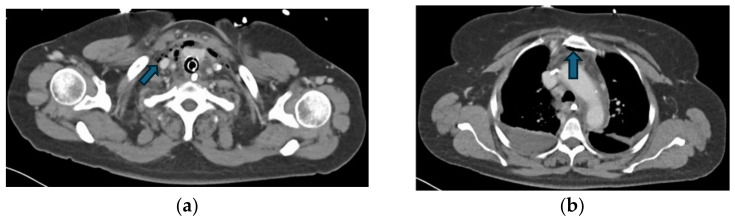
CT findings of the same patient presented in Figure 2, demonstrating the progression of DNM. (a) Gas inclusions (blue arrow) extending along the platysma muscle and thyroid gland into the anterior mediastinum; (b) Gas inclusions (blue arrow) posterior to the manubrium sterni with right sided pleural effusion.
